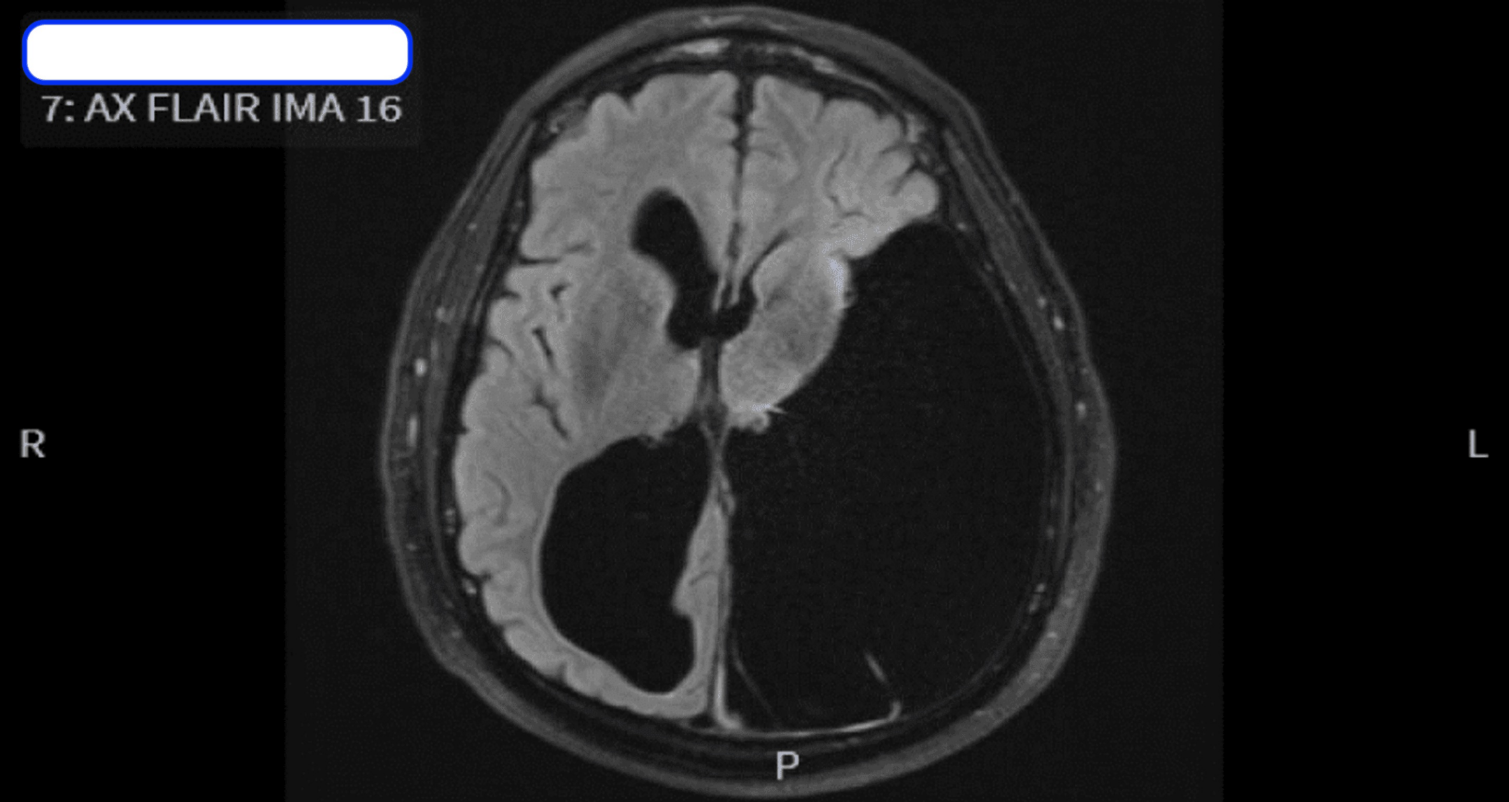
MRI brain imaging displayed a large porencephalic cyst in the left temporal, frontal, and parietal occipital lobes, communicating with the left lateral ventricle, and a chronically dilated right lateral ventricle.
